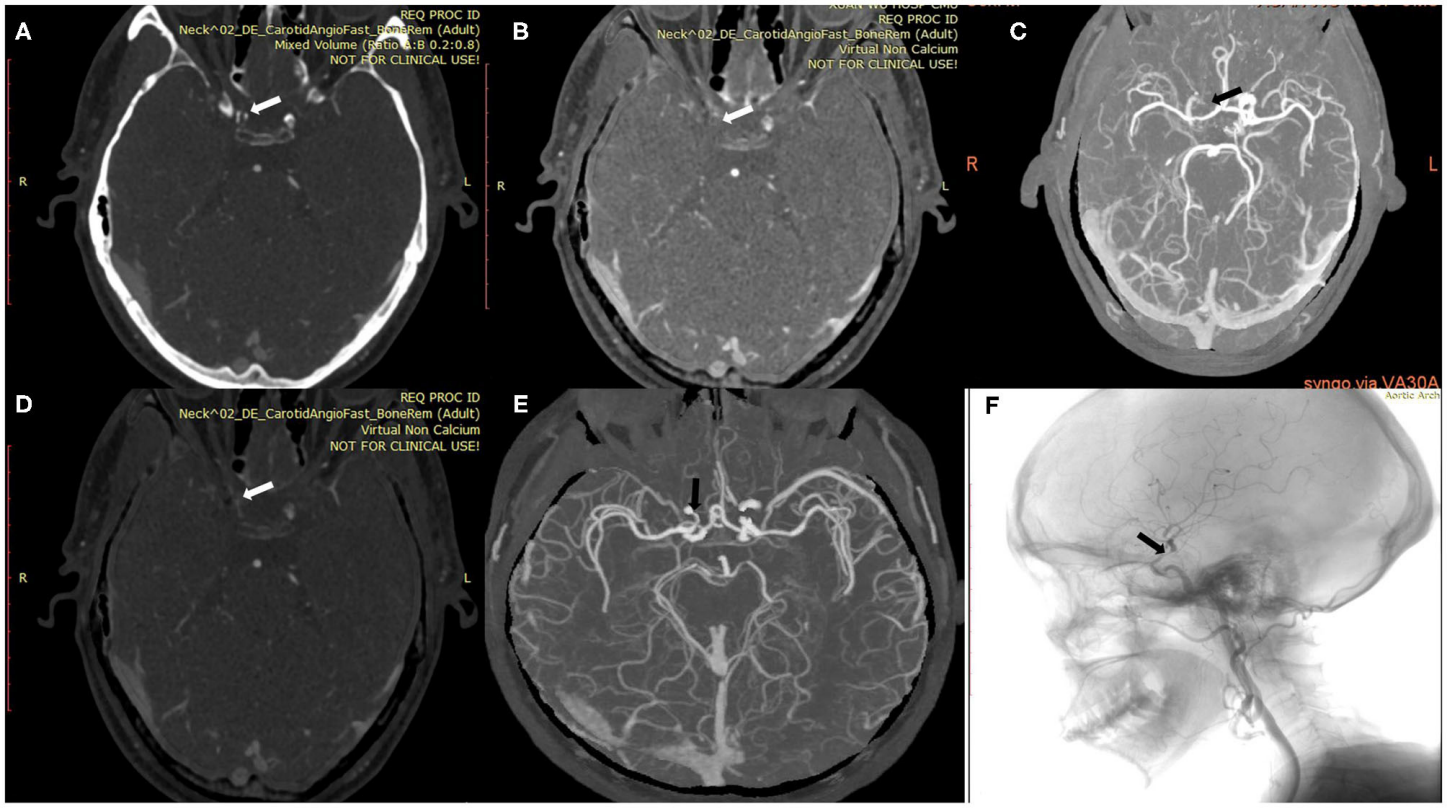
A 54-year-old man with calcified severe stenosis on the right intracranial internal carotid artery (ICA). (A) Axial conventional CTA at the location of calcified carotid artery stenosis (arrow). (B,C) Axial VNCa 1 and MIP at the location of calcified carotid artery stenosis (arrow). (D,E) Axial VNCa 2 and MIP at the location of calcified carotid artery stenosis (arrow). (F) DSA in sagittal projection indicated the severe stenosis (arrow). VNCa 2 had good consistency with DSA, and VNCa 1 tend to overcome the severe stenosis to occlusion.
